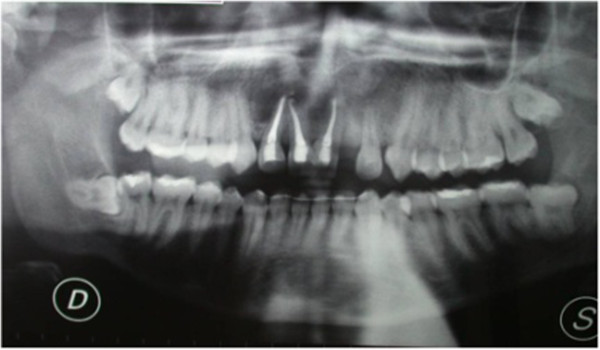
Persistence of periapical radiolucency at six months control.
